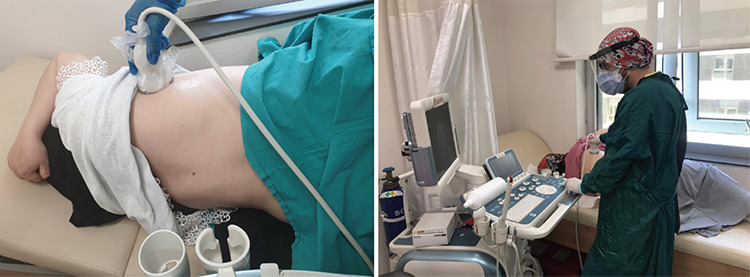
Lung ultrasound examination following a fetal assessment in supine and sided positions
